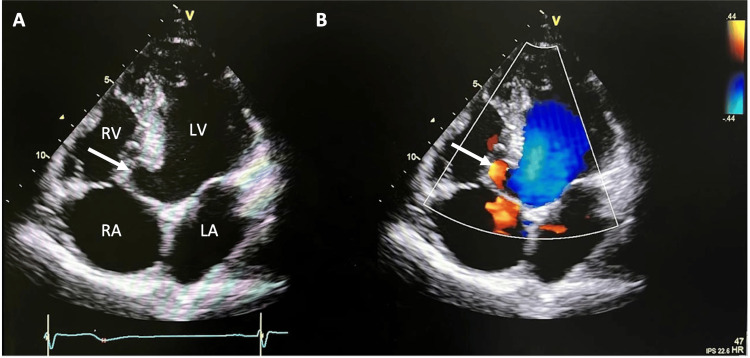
Transthoracic apical four-chamber view echocardiography and Color Doppler. Transthoracic apical four-chamber view echocardiography showing the membranous interventricular septal aneurysm (arrow) measuring 21 × 19 mm (neck width and depth, respectively) (A). Color Doppler overlying the aneurysm revealed no evidence of shunting (B). LA: left atrium; LV: left ventricle; RA: right atrium; RV: right ventricle.
